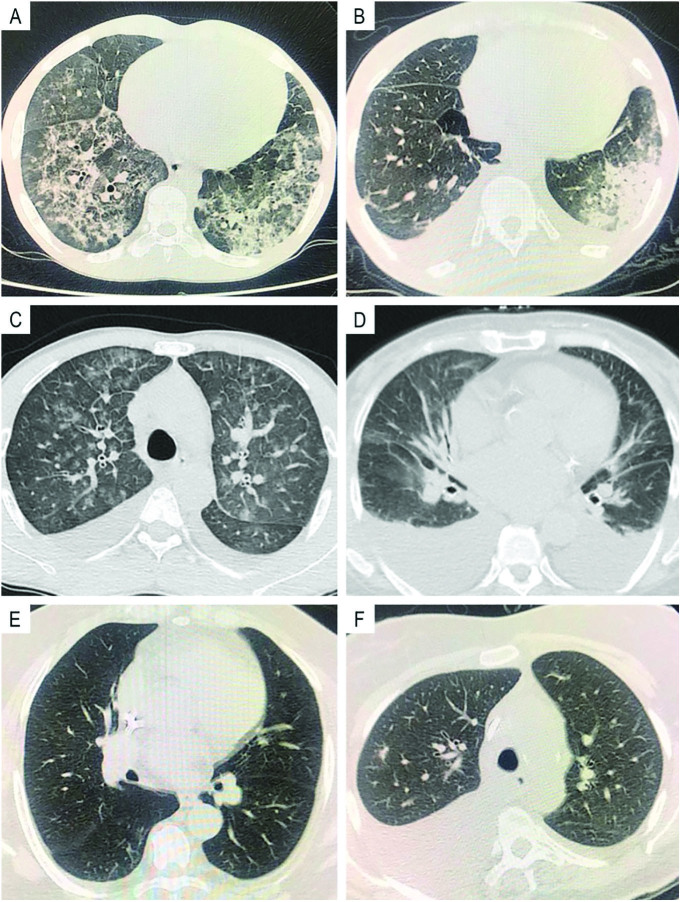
Computed chest tomography patterns in COVID-19. (A) Typical COVID-19: peripheral and bilateral ground glass opacity, consolidation and visible intralobular lines (“crazy-paving”); (B) Indeterminate: bilateral ground glass opacity especially in inferior lobes and pleural effusion; (C) Atypical: central ground glass opacity, bronchovascular bundle thickening and interlobular septal thickening, suggesting interstitial pulmonary edema; (D) Similar to image C plus pleural effusion and cardiomegaly, suggesting hydrostatic pulmonary edema; (E) Atypical: isolated consolidation with areas of ground glass opacities; (F) Negative: No CT features that suggest pneumonia.
